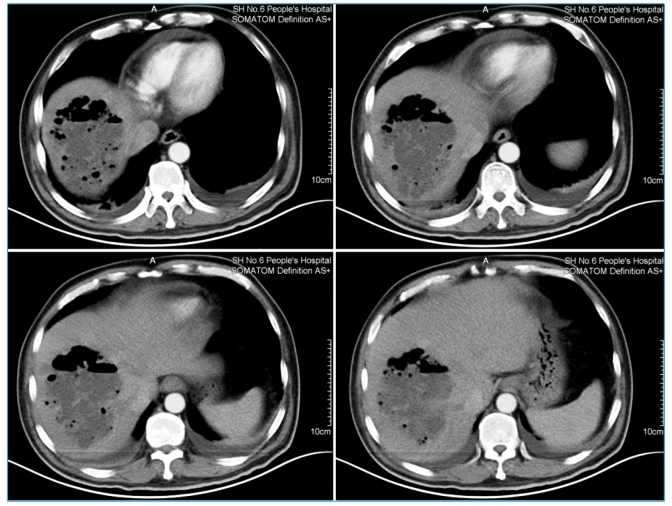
Contrast-enhanced CT scan of the abdomen showing a large abscess (12 cm×11 cm×9 cm) with gas formation in the right lobe of the liver.
